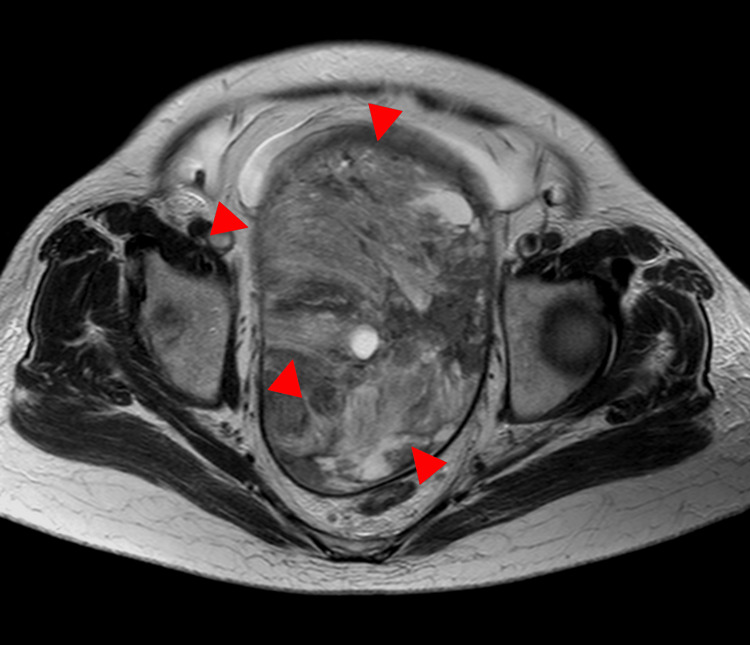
This is the same patient as shown in Figure 11. On the axial T2-weighted image, the solid component demonstrates heterogeneous signal intensity with a layered appearance, consistent with the mille-feuille sign (arrowhead).
